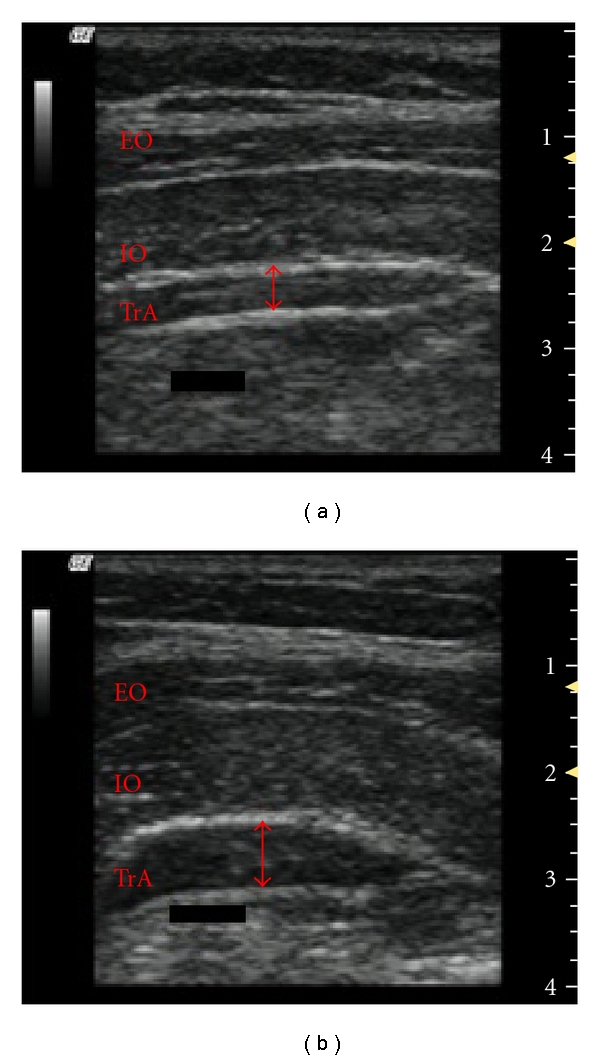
Ultrasound image of the TrA and calculation of TrA activation ratio. Resting image is on (a) and contracted image is on (b) (Activation ratio = TrA contracted (mm)/TrA rest (mm)). EO = external oblique; IO = internal oblique; TrA = transverse abdominis. The red arrow indicates the thickness of the TrA.
